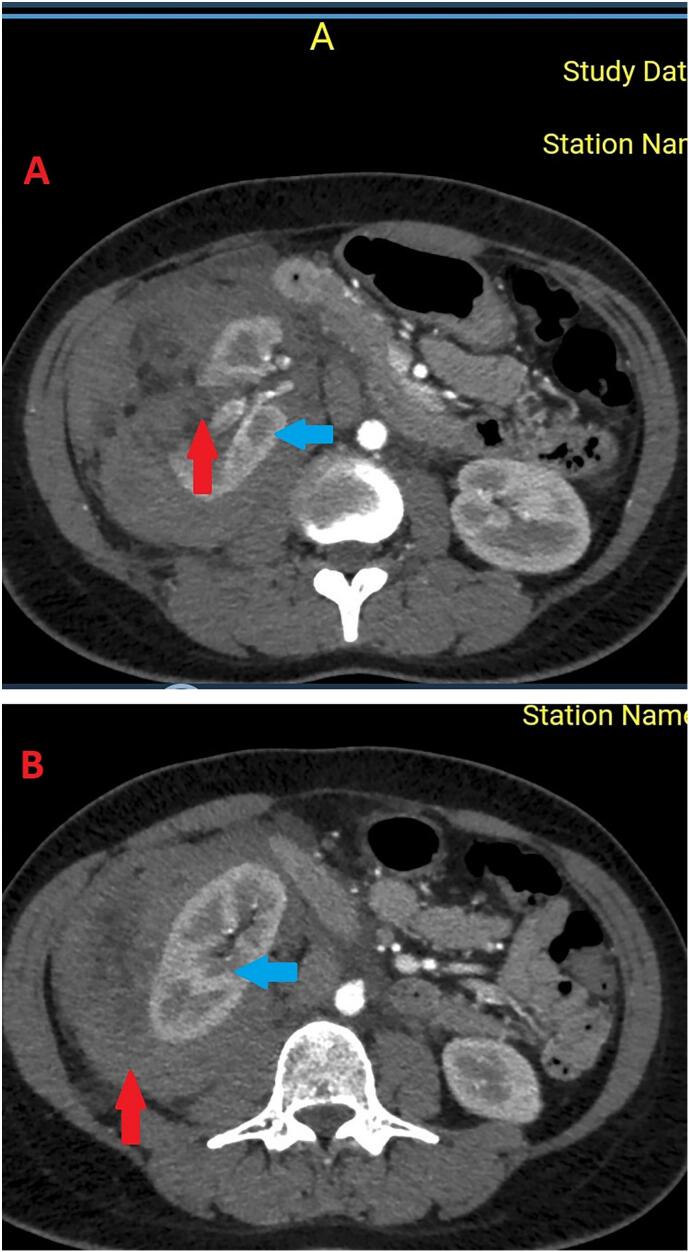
CT scan of abdomen with intravenous contrast axial view. A – Showing an exophytic lesion with a sentinel clot sign (red arrow) arrising from the right kidney (blue arrow). B – Showing a hematoma (red arrow) surrounding the right kidney (blue arrow). (For interpretation of the references to colour in this figure legend, the reader is referred to the web version of this article.)
